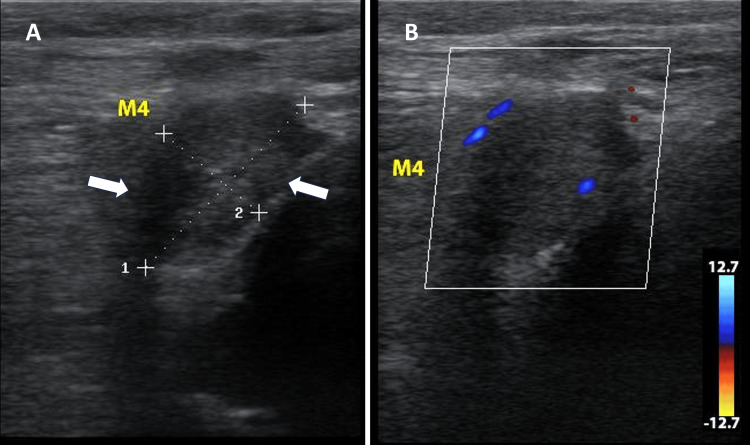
B-mode and color Doppler ultrasound images of M4 (A) B-mode ultrasound image of M4, a mass in the popliteal fossa, measuring 2.39 cm (dotted line 1) x 1.30 cm (dotted line 2). (B) Color Doppler ultrasound image of M4, showing vascularization within the mass in the popliteal fossa (blue and red coloration)
